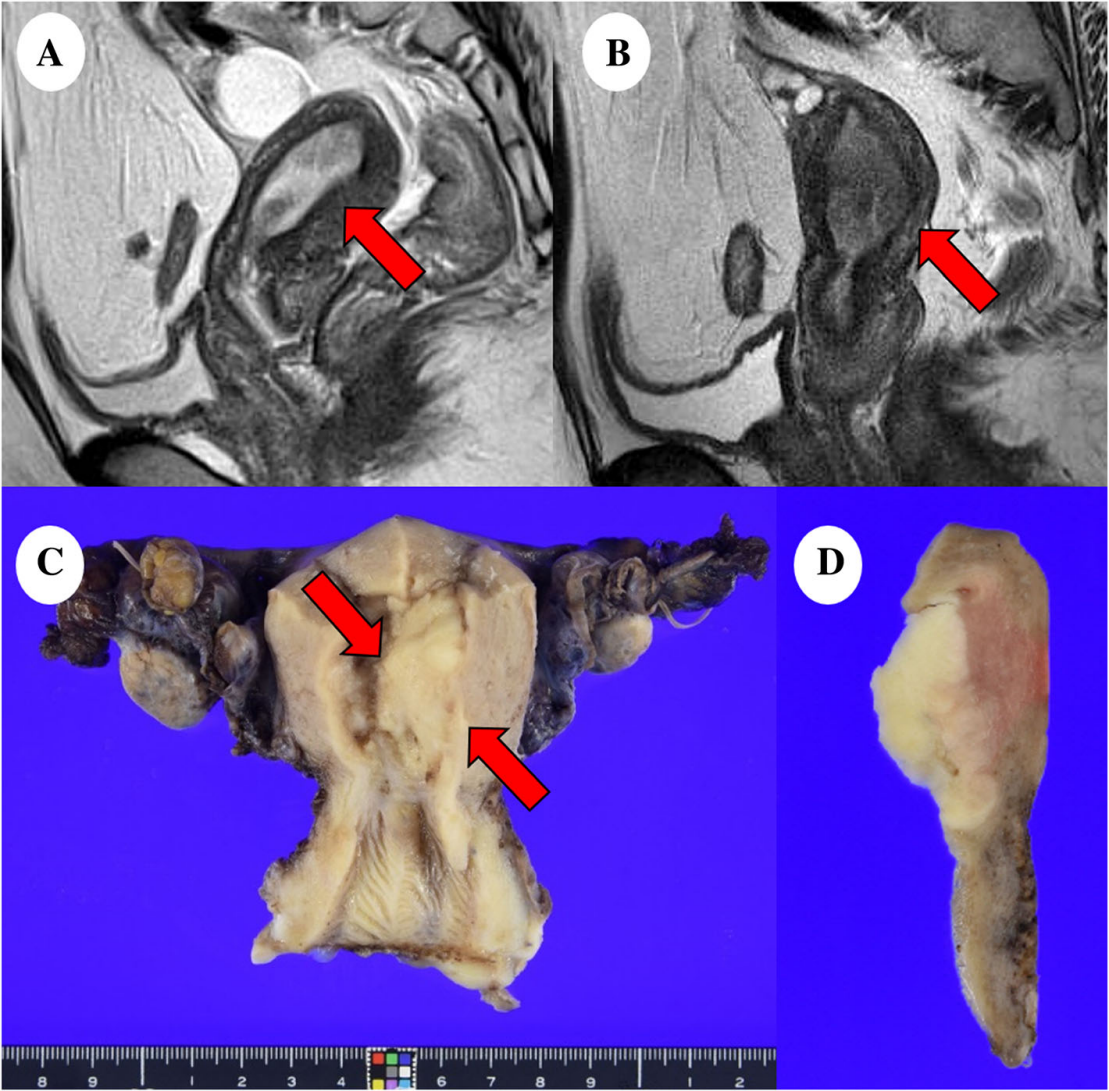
Magnetic resonance imaging and macroscopic analysis: (a) T2-weighted image of the initially diagnosed tumor (red arrow). (b) T2-weighted image of the recurred tumor (red arrow). (c) The endometrial mass (red arrows) was 40 × 23 mm-sized in the left wall of the uterine body and (d) had yellow-whitish cut surface
